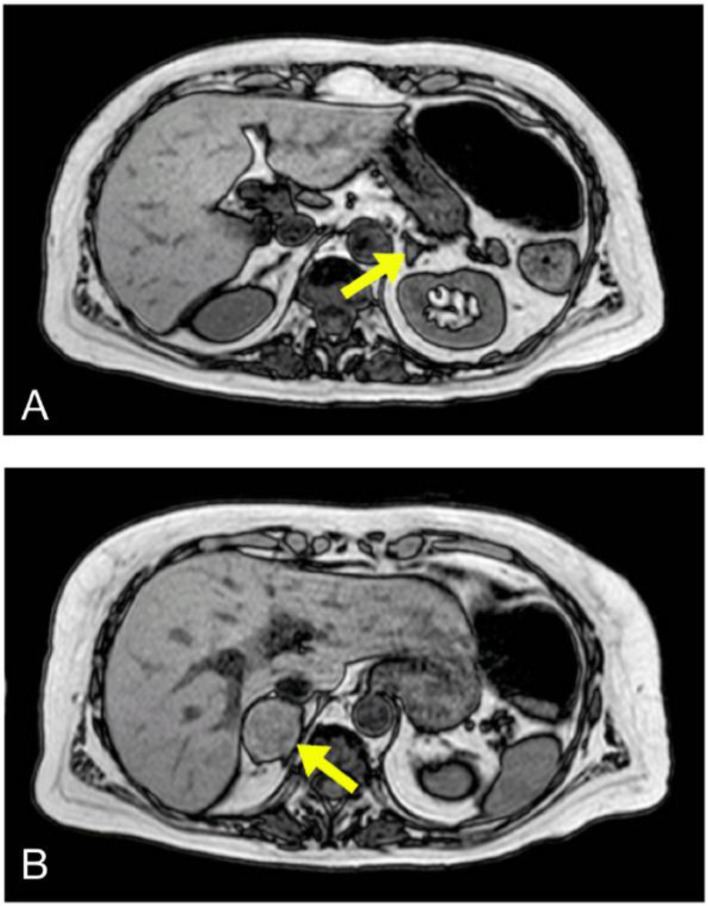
In panel (A) the MRI image of left PCC (6 mm) and in panel (B) the MRI image of right PCC (40 mm).
